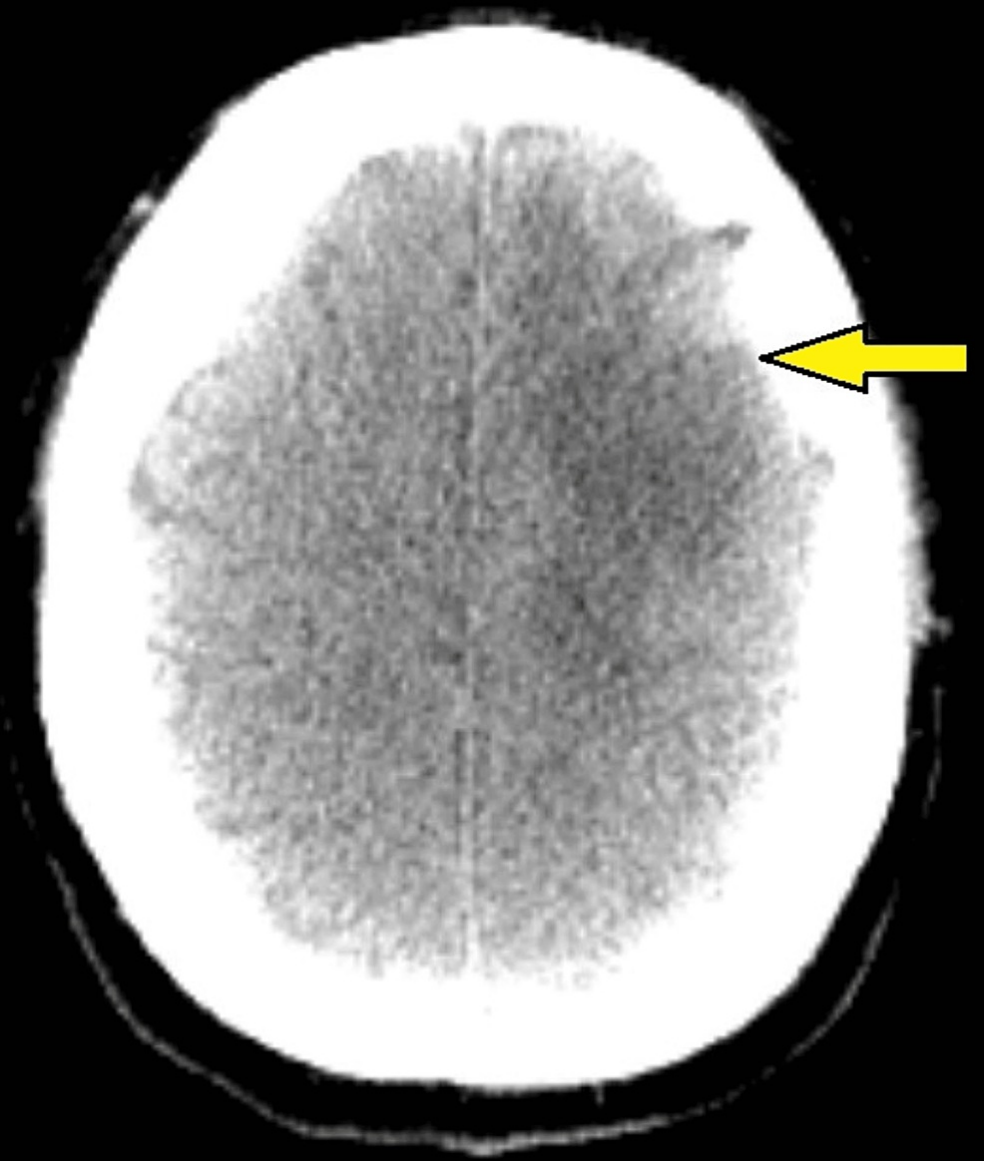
Postoperative CT after surgical exertion of a left frontal glioma. Axial plane. Notice the exerted part in the left frontal region of the cerebrum.
